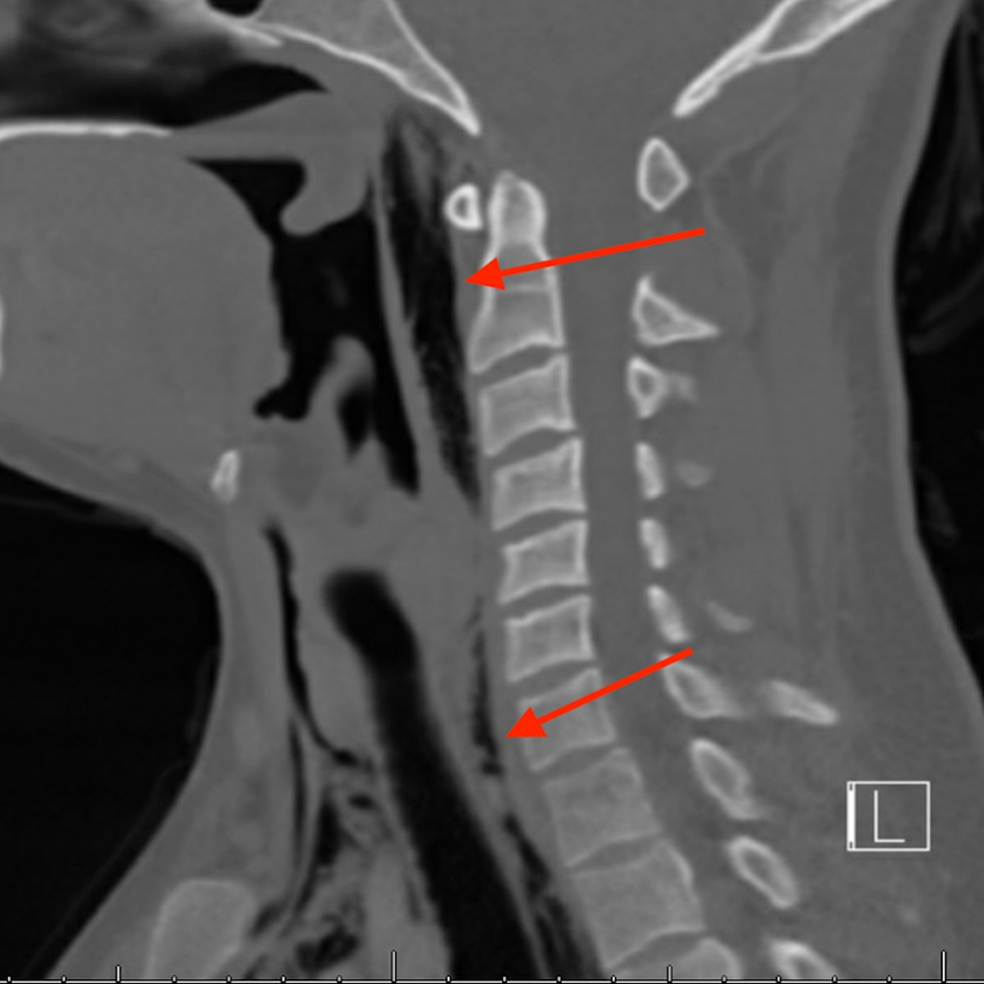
CT scan of the neck with intravenous contrast (sagittal section) showing gas tracking into the retropharyngeal space (red arrows) L - left
